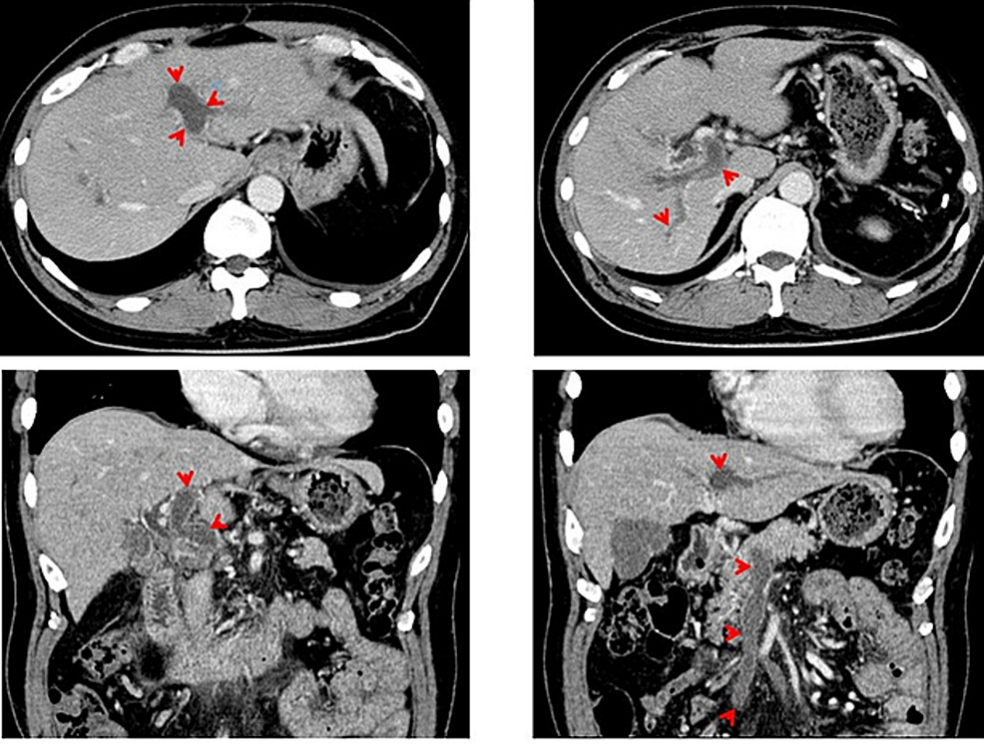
Abdominal computed tomography findings Upper row: The intra-hepatic portal vein was almost occluded by a thrombus (red arrow). Lower row, longitudinal image: The thrombus (red arrow) was also observed in the extra-hepatic portal vein (left) and the superior mesenteric vein (right) continuously (thrombus was also found in the intrahepatic portal vein).
